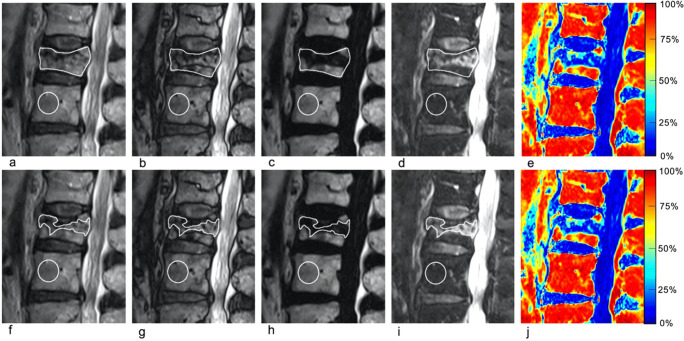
75-year-old woman with a benign vertebral compression fracture (VCF) (a–j). Sagittal thoracic spine MR images generated from a single FSE T2-weighted Dixon sequence include in-phase (a and f), out-of-phase (b and g), fat-only (c and h), water-only images (d and i), and fat fraction (FF) maps (e and j). Region of interest (ROI)1 (a–e) was drawn on the entire vertebra at the level of the VCF on (a) in-phase image and copy-pasted onto the (b) out-of-phase, (c) fat-only, (d) water-only images, and (e) fat fraction maps. ROI2 (f–j) was drawn on the area with high signal intensity on the (i) water-only image, and copy-pasted onto the (f) in-phase, (g) out-of-phase, (h) fat-only images, and (j) fat fraction maps. Control ROI (circle) placed on an adjacent healthy vertebra and is depicted on all images. In this benign VCF, signal drop, FF calculated from water-only and fat-only images, and FF from maps were ROI1: 50.4%/ROI2: 47.6%; ROI1: 51.4%/ROI2: 36.2%; and ROI1: 39.8%/ROI2: 31.3%, respectively
